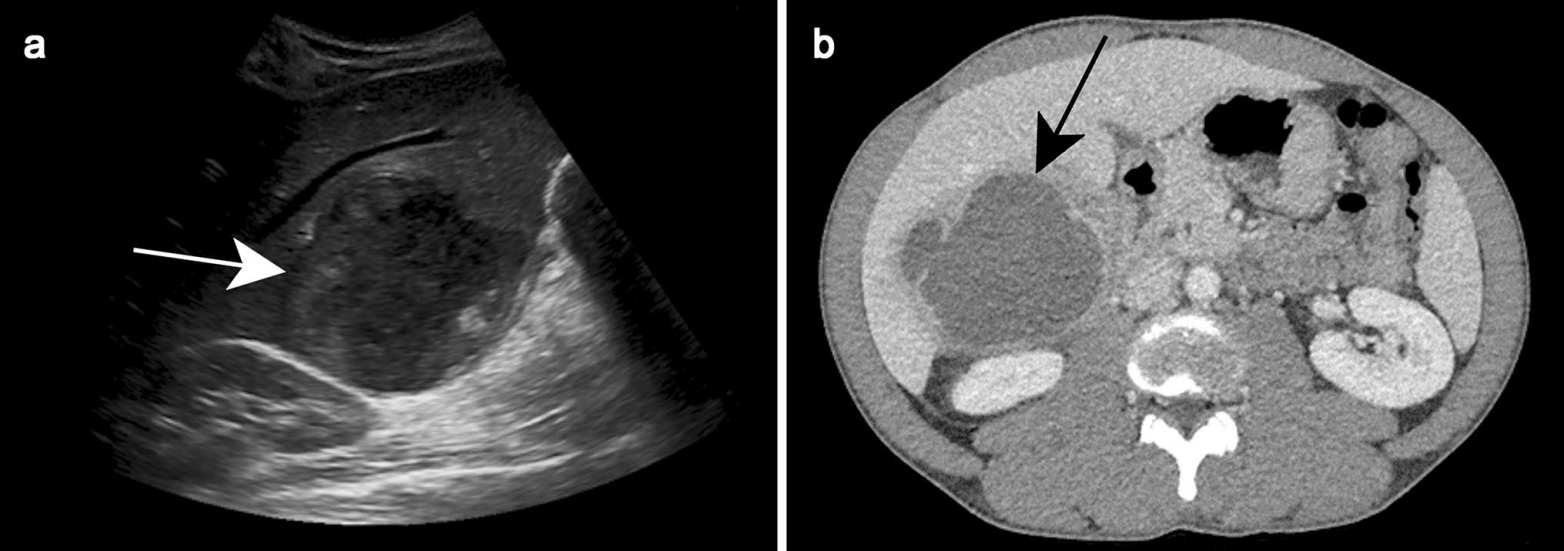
Amebic abscess in a 57-year-old male patient who presented with fever of unknown origin and right upper abdominal pain with a recent history of travel to Africa. Ultrasound a demonstrates a large relatively well-delineated lesion with a heterogeneous solid-appearing content (white arrow). Axial contrast-enhanced CT shows the “double target sign” (black arrow)
